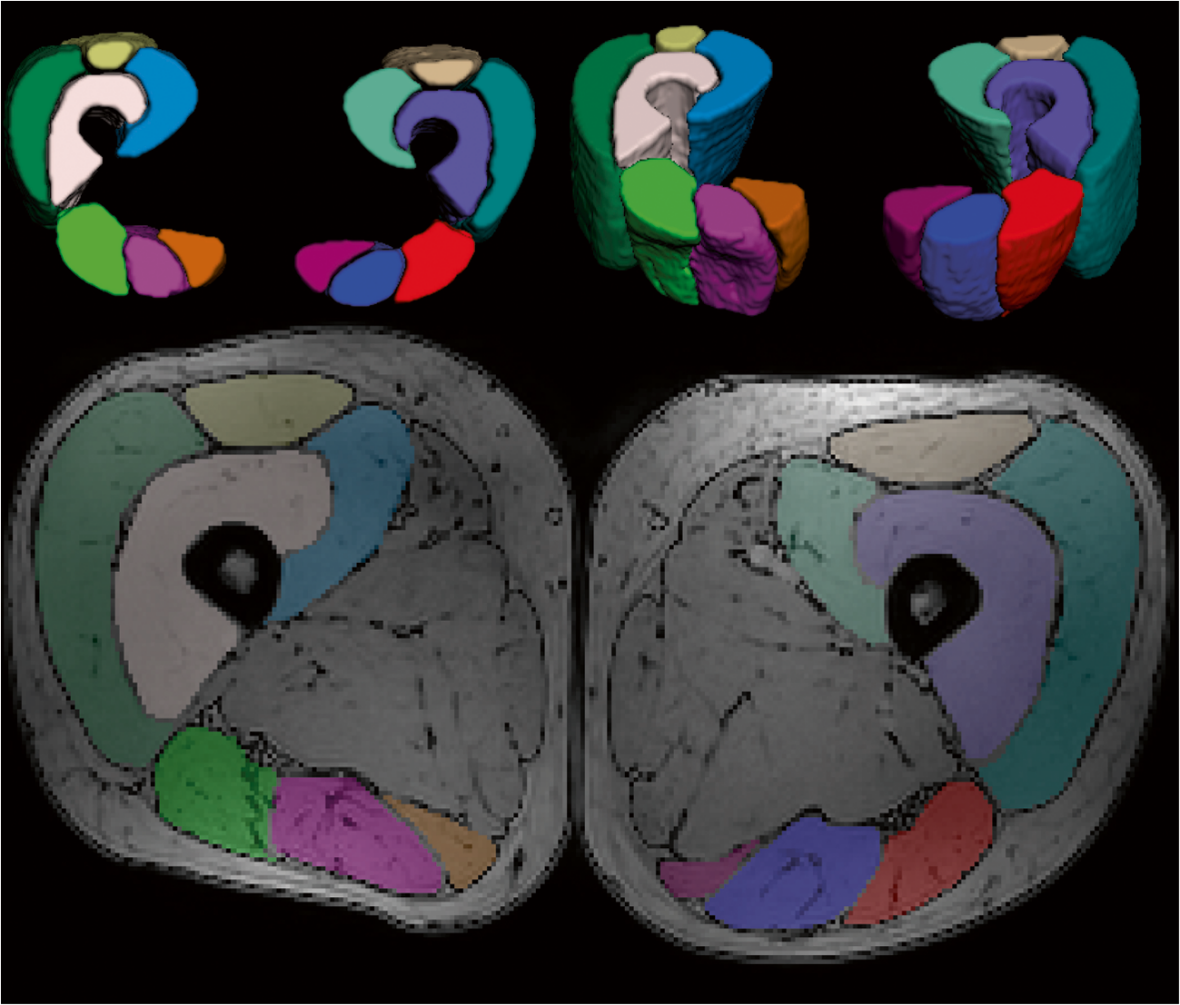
a Representative axial proton density–weighted MRI with overlaid muscle segmentations in color. b 3D volume rendering of segmented muscles in the center 20 slices of the upper leg
